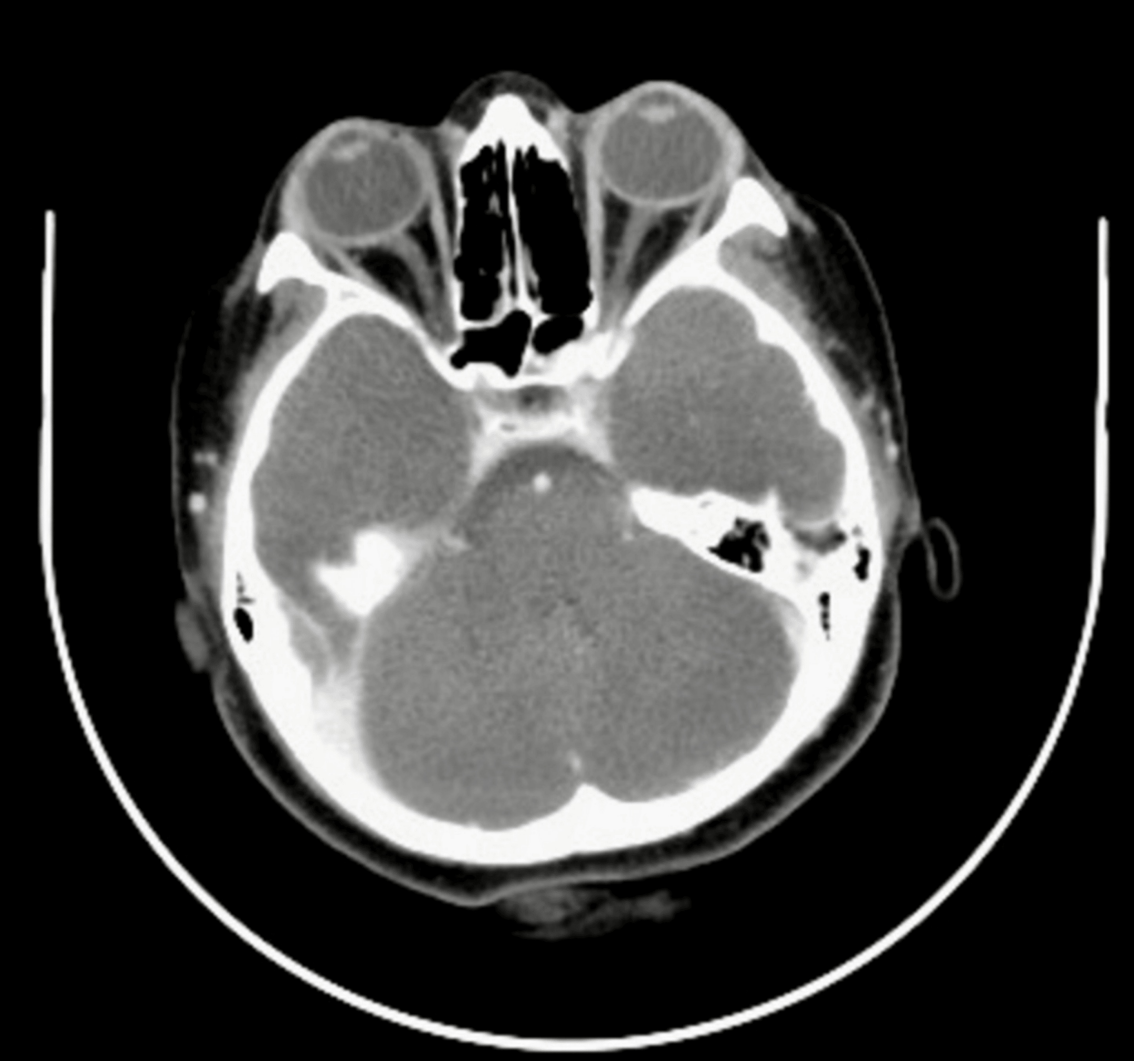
Repeat CT scan showing resolution of ocular proptosis and inflammation
